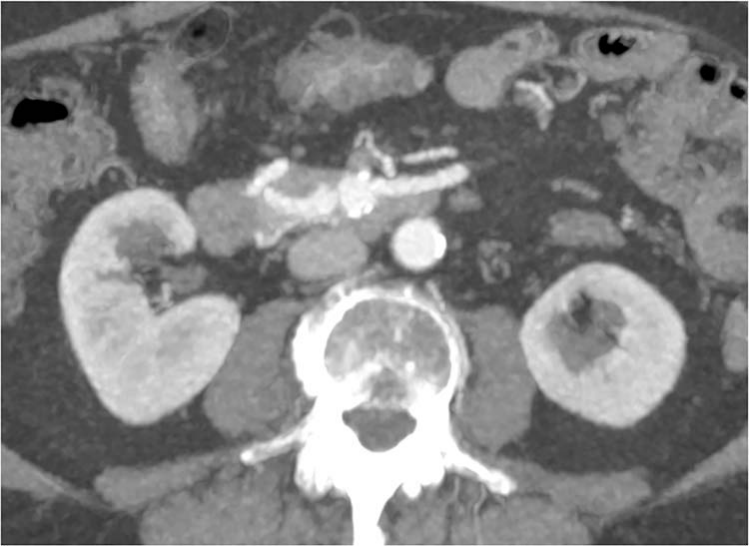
Multiphase CT pancreas, axial view demonstrating abnormal communication between the CA and the SMA.
